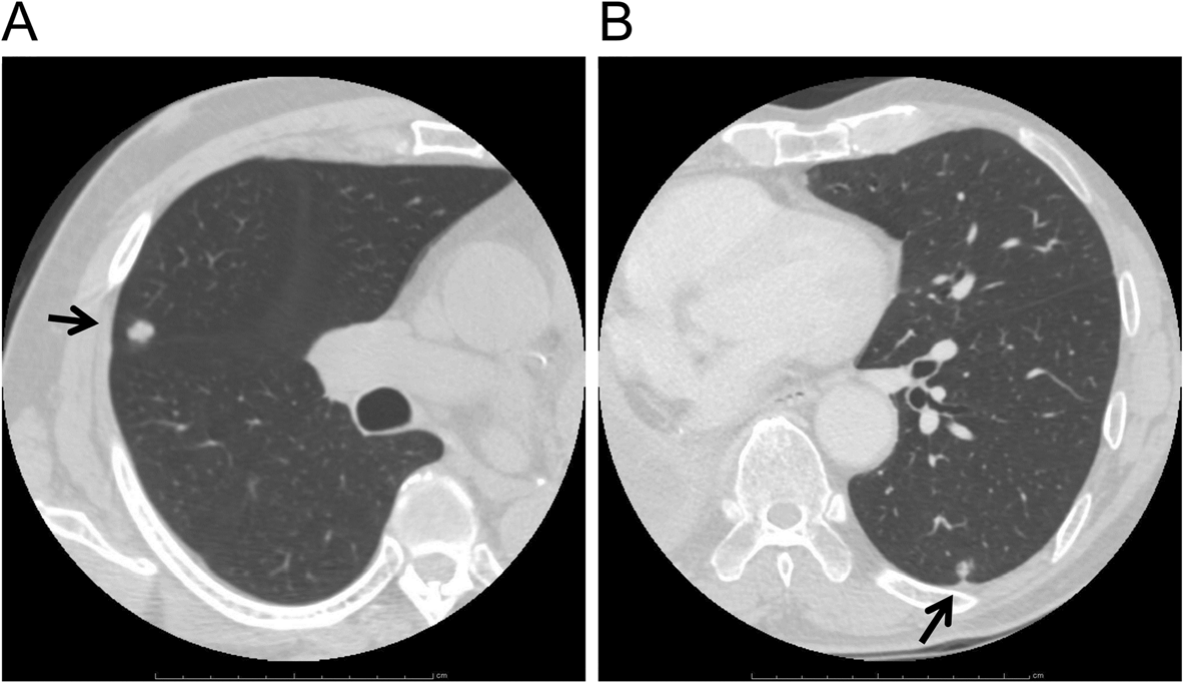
Preoperative computed tomography images The arrows indicate the tumor location. In one case, the tumor just inferior of the pleura at the right S4 lung segment is visualized in the horizontal plane (a). In the second case, the tumor just inferior of the pleura at the left S10 lung segment is visualized in the horizontal plane (b).
